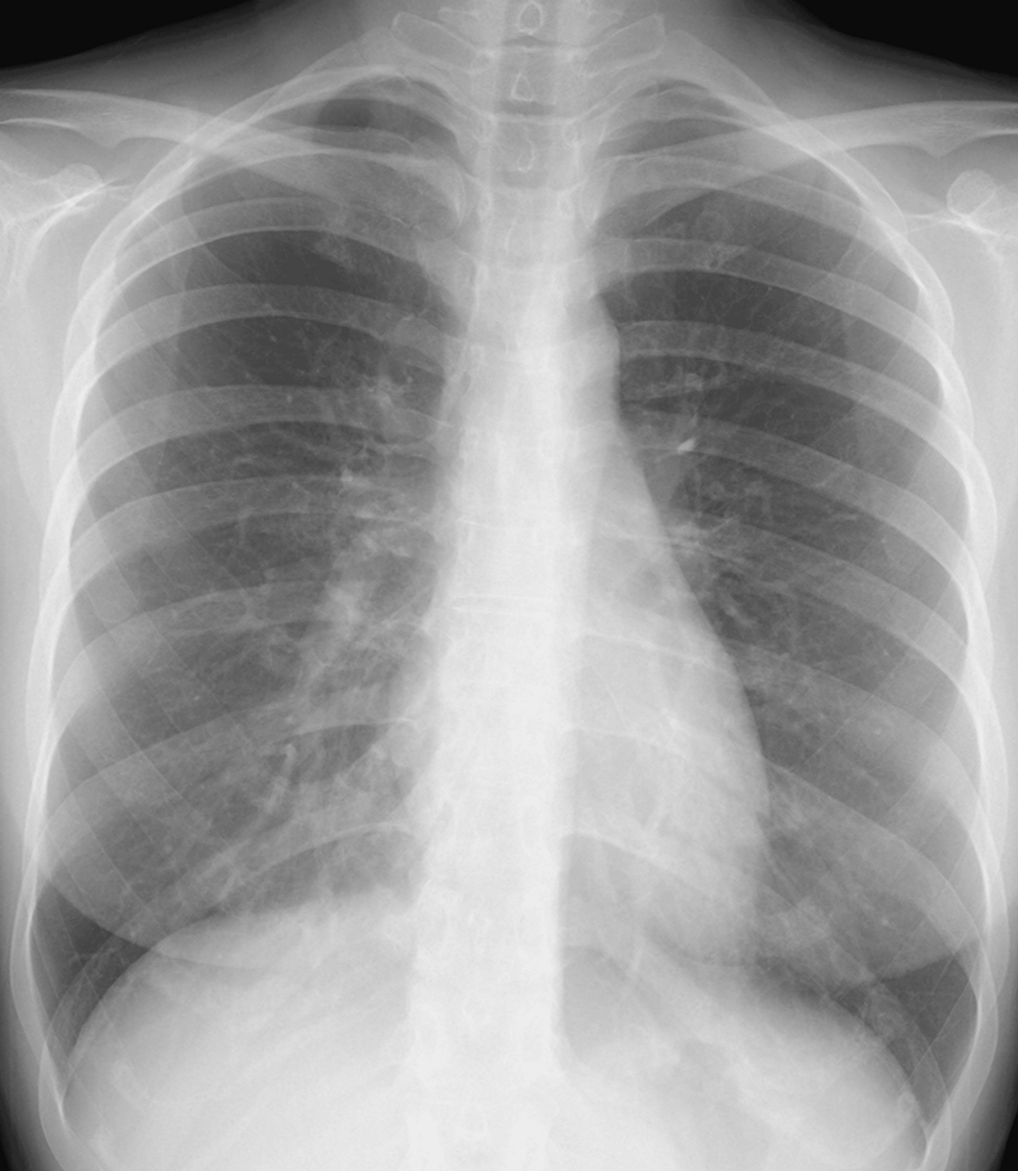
A chest radiography at the initial visit. The chest imaging was a posterior-anterior view. Pneumonia was not found on chest radiography performed at the initial visit.
